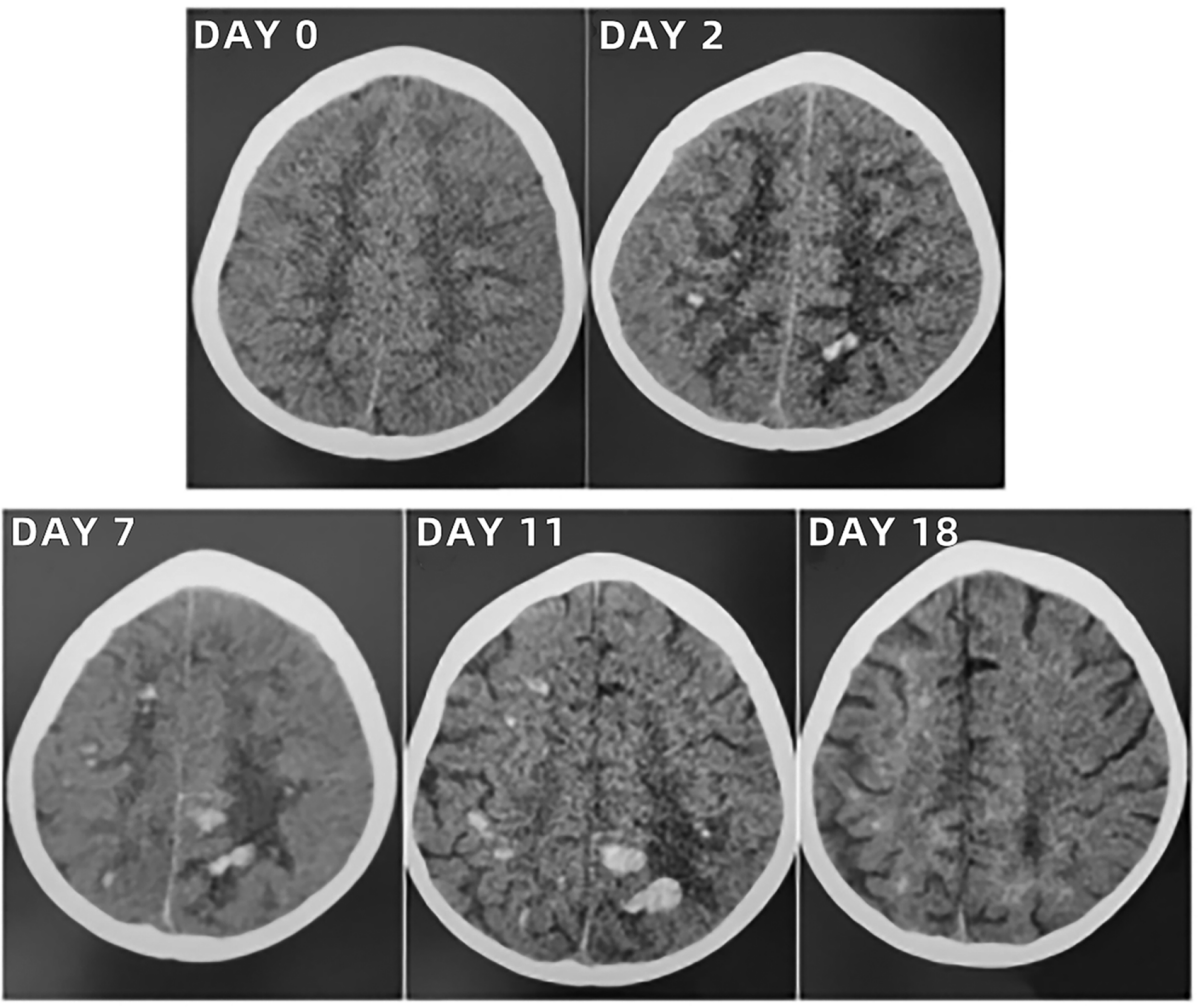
Brain computed tomography scans of the toddler during hospitalization.
